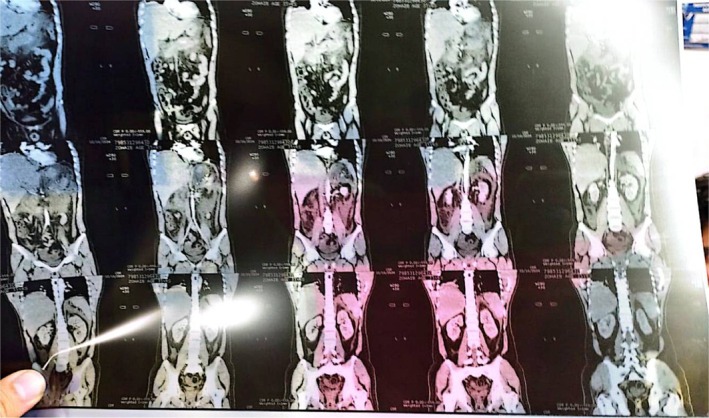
Coronal Reformatted CT Scan Demonstrating the longitudinal extent of pancreatic swelling and the presence of mild to moderate abdomino‐pelvic ascites.
